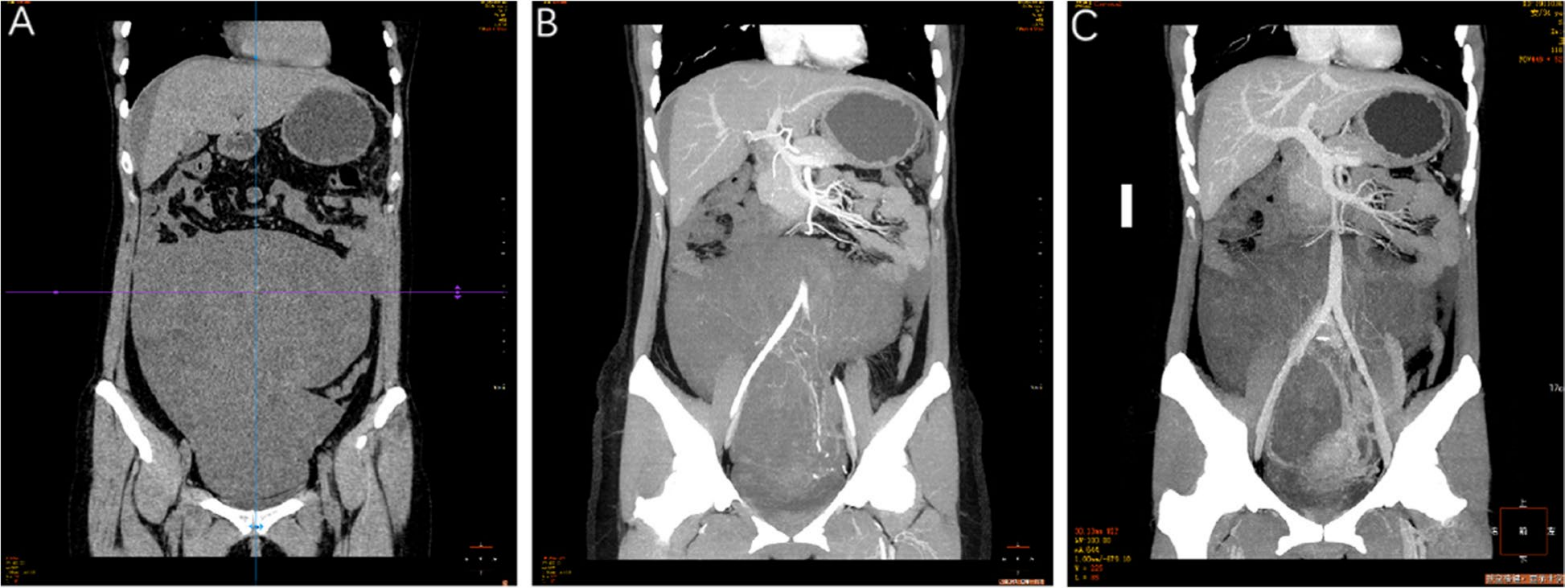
The computed tomography scan of pelvic cavity. A (CT plain scan) Huge mass occupying the pelvic cavity, the size of about 8.1*22.3*25.8 cm, its internal density is low, slightly mixed. B (CT enhancement (venous phase)) The enhancement was not obvious, and the huge pelvic occupying vein joined the left ovarian vein. C (CT enhanced (arterial phase)) Huge pelvic mass supplied by the left ovarian artery
